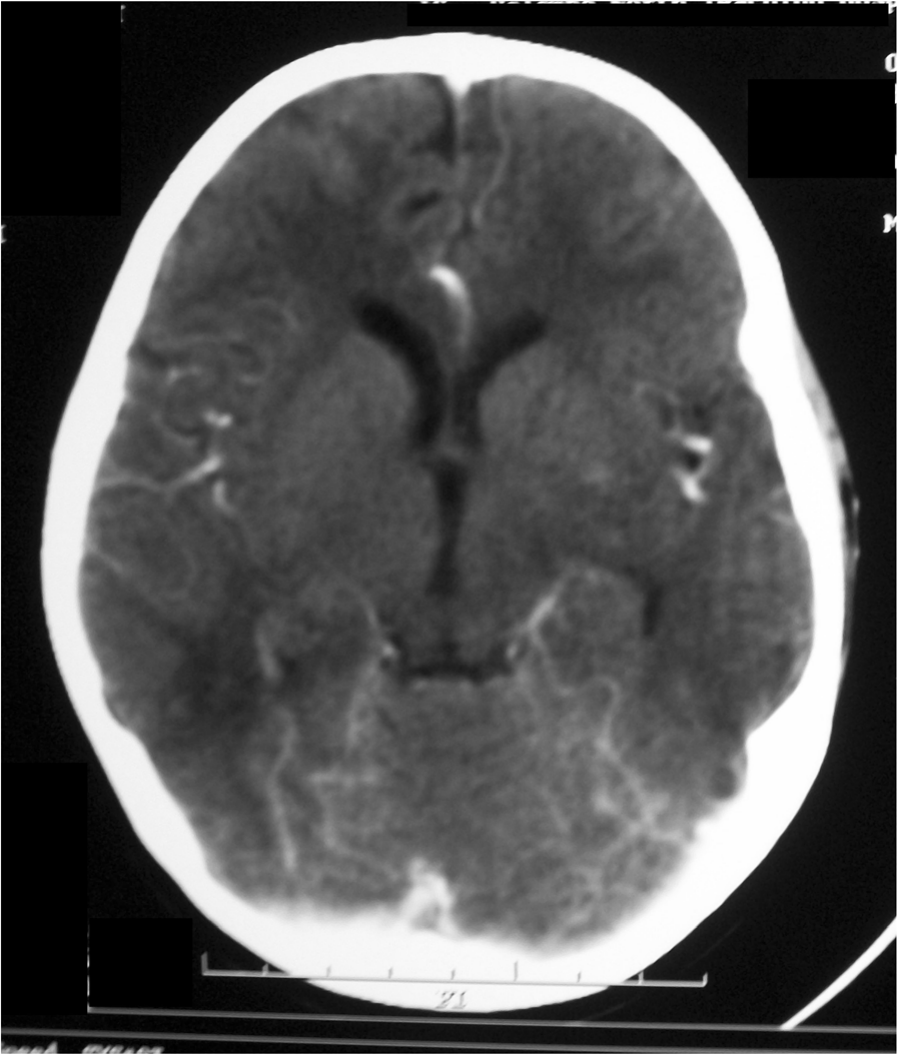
Contrast-enhanced computed tomography scan of brain showing bilateral symmetrical paramedian hypodensities involving cortical and subcortical white matter seen in posterior parietal regions suggestive of posterior reversible encephalopathy syndrome.
